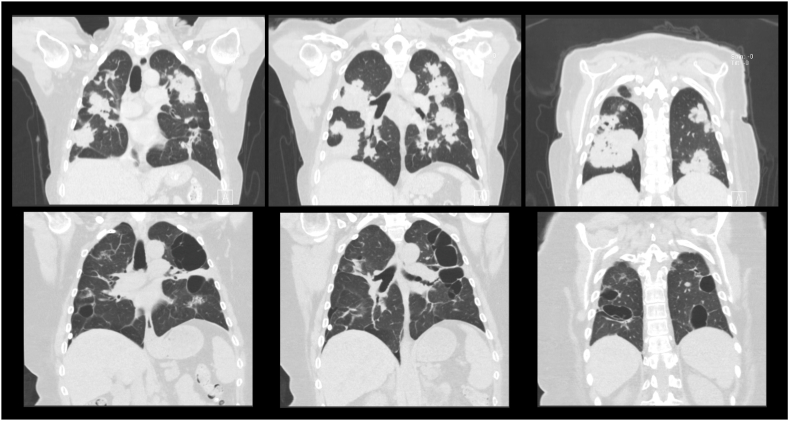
The figure depicts two coronal CT scans. The top row is an initial CT scan demonstrating significant tumor burden, preceding immunotherapy. The bottom row is from a CT scan taken after initiation for monitoring of tumor resorption. Slices are anatomically paired, moving anterior to posterior going left to right. This matching highlights the exact location of metastatic lesions (top row), and the appearance of cystic airspaces only where tumor was previously located (bottom row).
